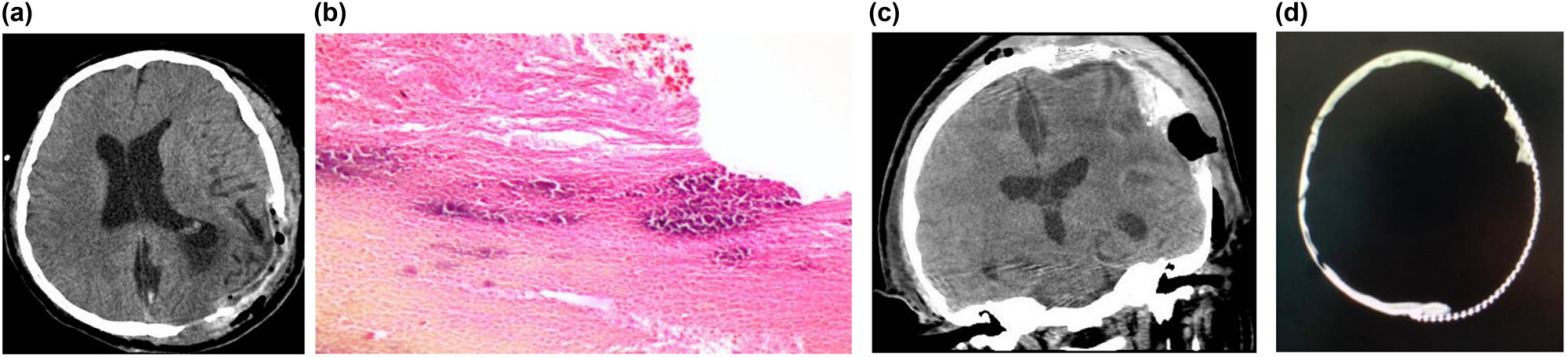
Postoperative examination. (a) CT detection on the first day after surgery. The lesion was completely removed, and the brain tissue was restored. (b) Postoperative pathology. (c) CT detection on the third day after surgery. A small amount of epidural hematoma in the left frontal area occurred, which was conservatively treated. (d) CT detection after cranioplasty at 6 months after surgery.
